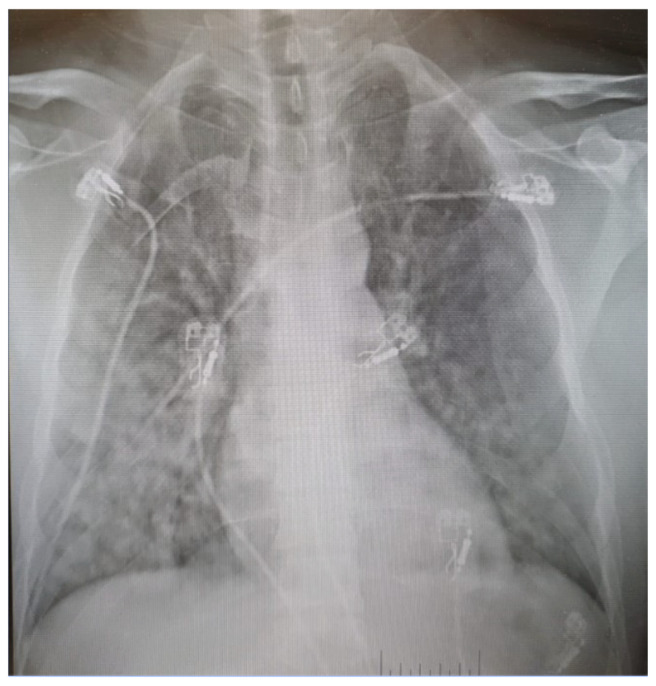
Initial assessment chest X-Ray.
